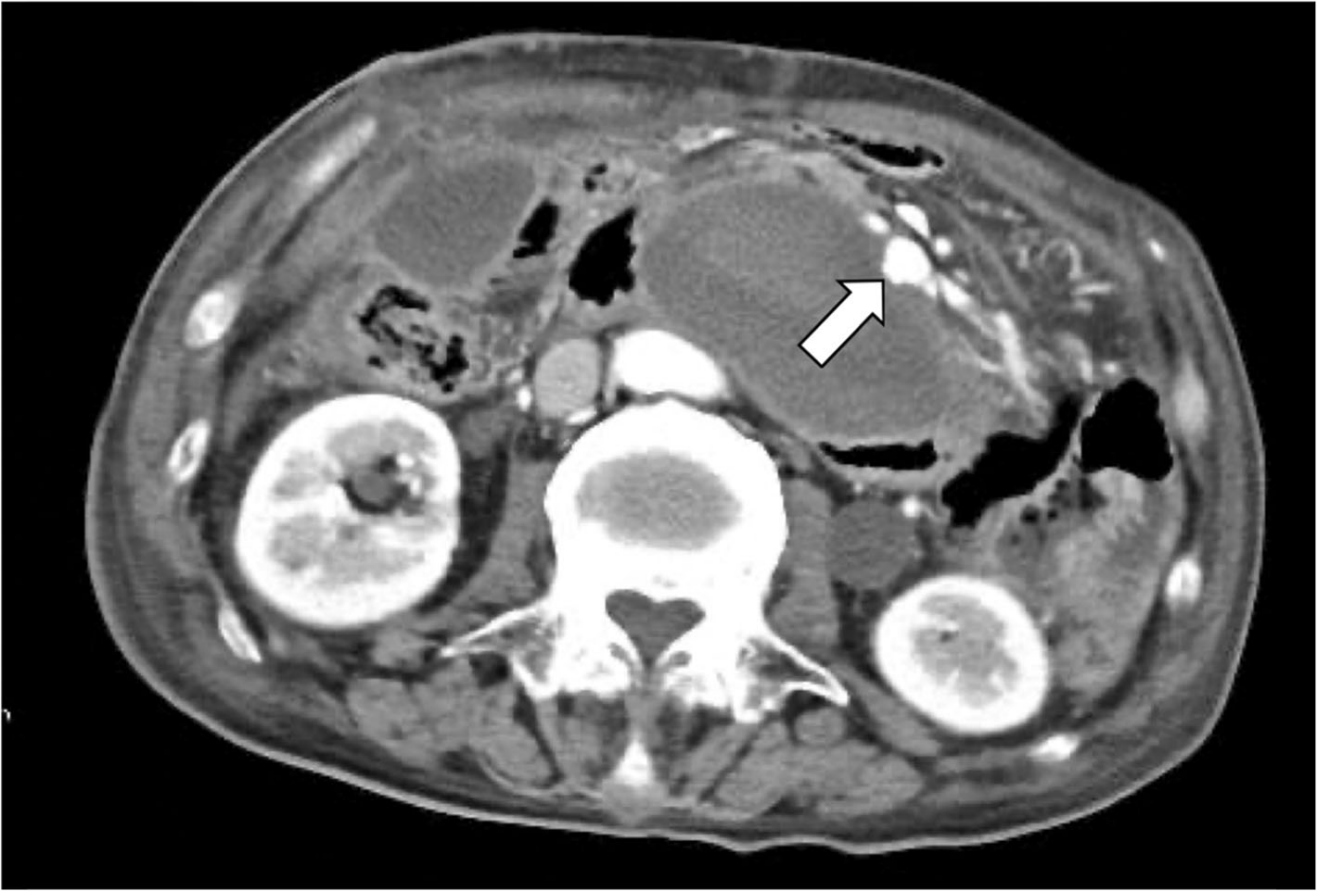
Computed tomography after first vomiting showed extravasations from the untreated anterior inferior pancreaticoduodenal artery aneurysm (shown by arrowheads). An untreated anterior inferior pancreaticoduodenal artery aneurysm appeared to be rounded (13 × 11 mm)
